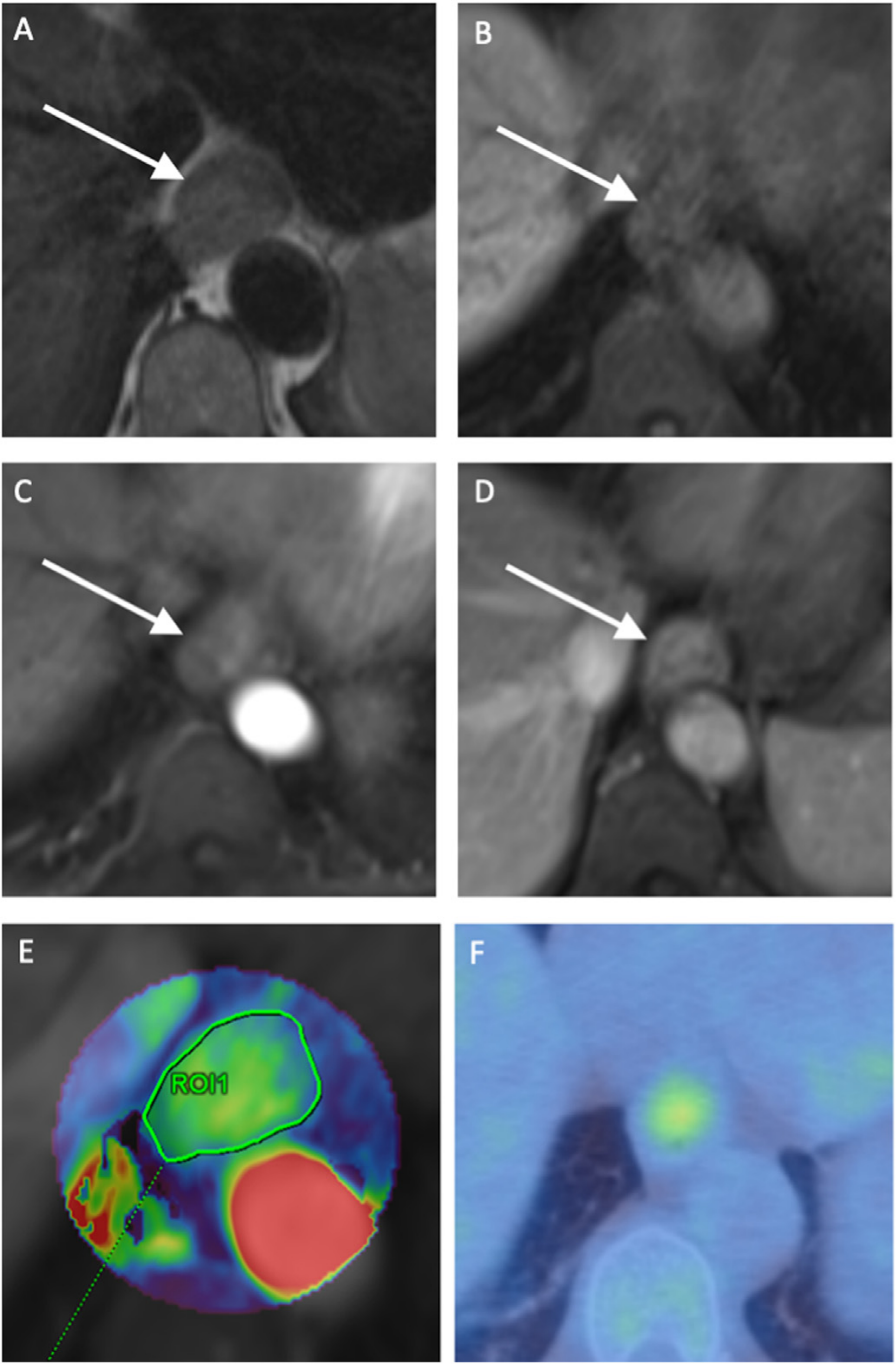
75-year-old with a lower esophageal adenocarcinoma who had a poor response to neoadjuvant therapy (TRG 4). Axial T2-weighted MRI image (A) demonstrates the primary tumor. Axial pre-contrast T1-weighted MRI image (B), axial arterial phase T1-weighted MRI image (C) and axial portal-venous phase T1-weighted MRI image (D) show early tumor enhancement with washout. Corresponding peak enhancement map (E) and ^18^F-FDG PET/CT (F) shows higher peak enhancement of 0.33 mmol L^−1^ and lower tumor metabolic activity, SUV_max_ of 5.7, respectively.
